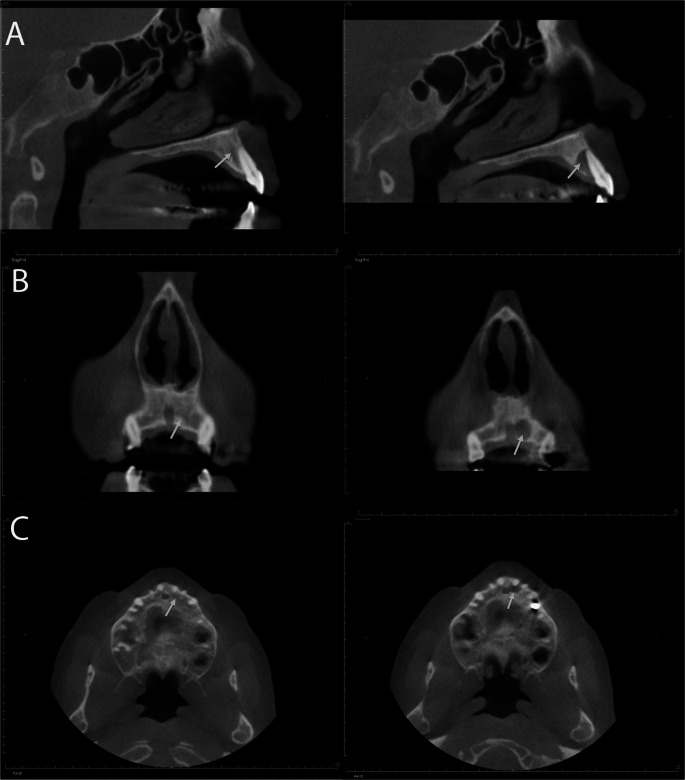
Radiographic findings of an intraosseous hair-induced cyst-like lesion between teeth #9 and #10. The region of interest from November 2023 (left-sided) and February 2025 (right-sided) demonstrated no evidence of pathosis found in November 2023 slices compared to the appearance of hydraulic-appearing, hypodense entity in February 2025. (Panel A) Sagittal view, (Panel B) Coronal view, and (Panel C) Axial view
